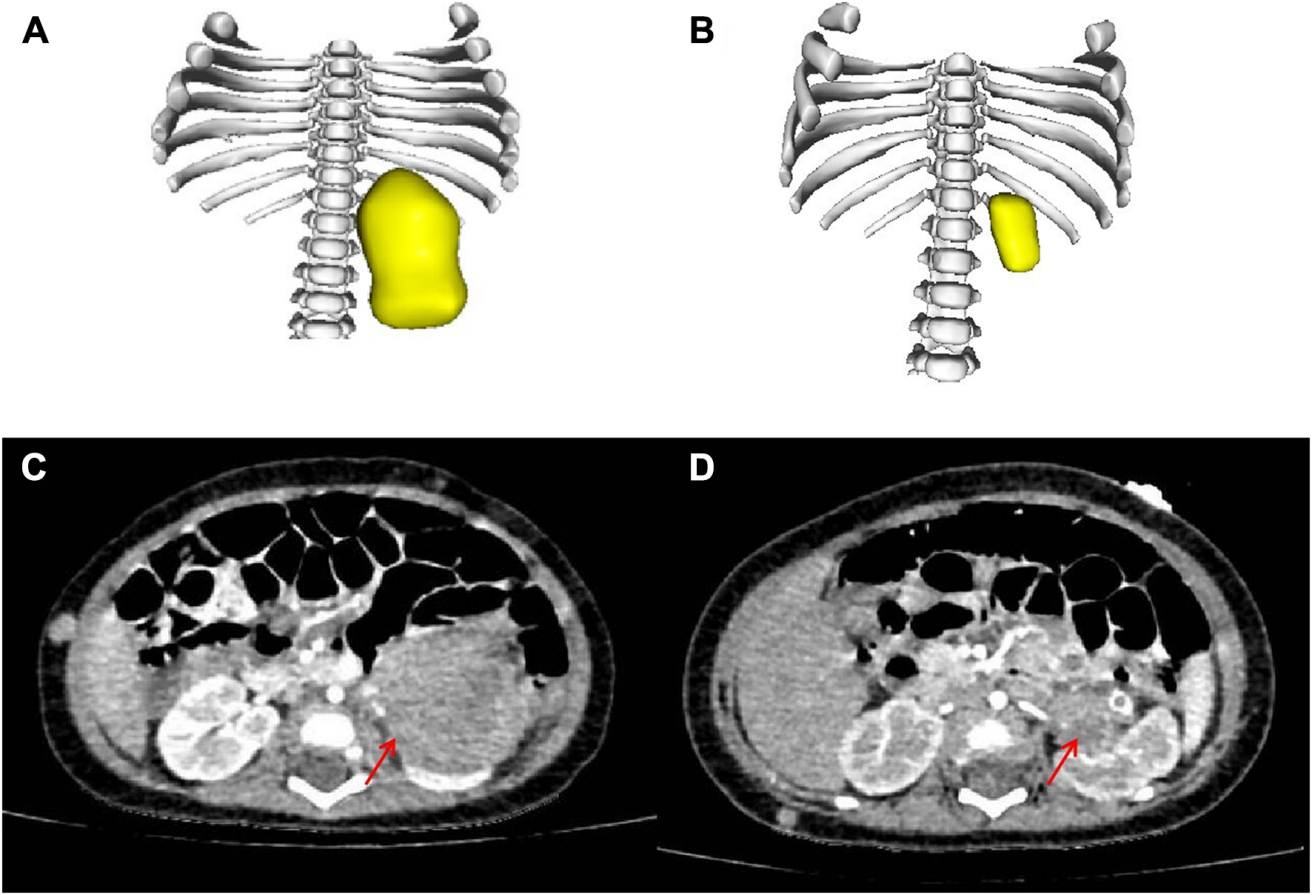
Evaluation of response to neoadjuvant chemotherapy in one patient with ACC. A, B 3D reconstruction before (A) and after (B) neoadjuvant chemotherapy in a 4-month-old patient with stage IV ACC. C, D Enhanced CT before (C) and after (D) neoadjuvant chemotherapy. Location of the primary tumor focus (arrow)
